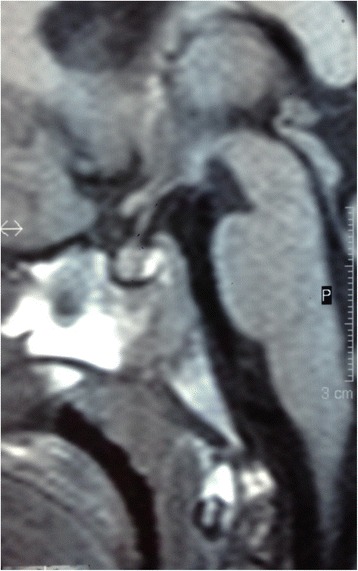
Magnetic resonance imaging. Sagittal and coronal precontrast T1-weighted image of the patient’s pituitary gland showing normal anterior and posterior lobes, with homogeneous enlargement and convex upper surface of the gland
